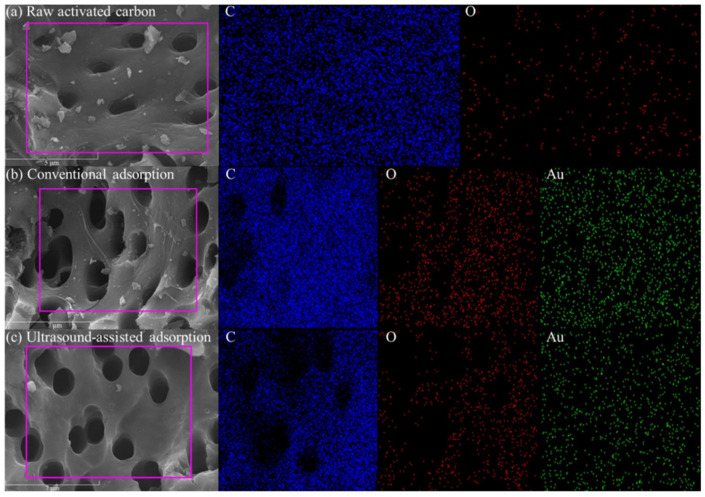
SEM images and main element distribution for raw activated carbon (a), activated carbon after conventional adsorption (b), and after ultrasound-assisted adsorption (c).
